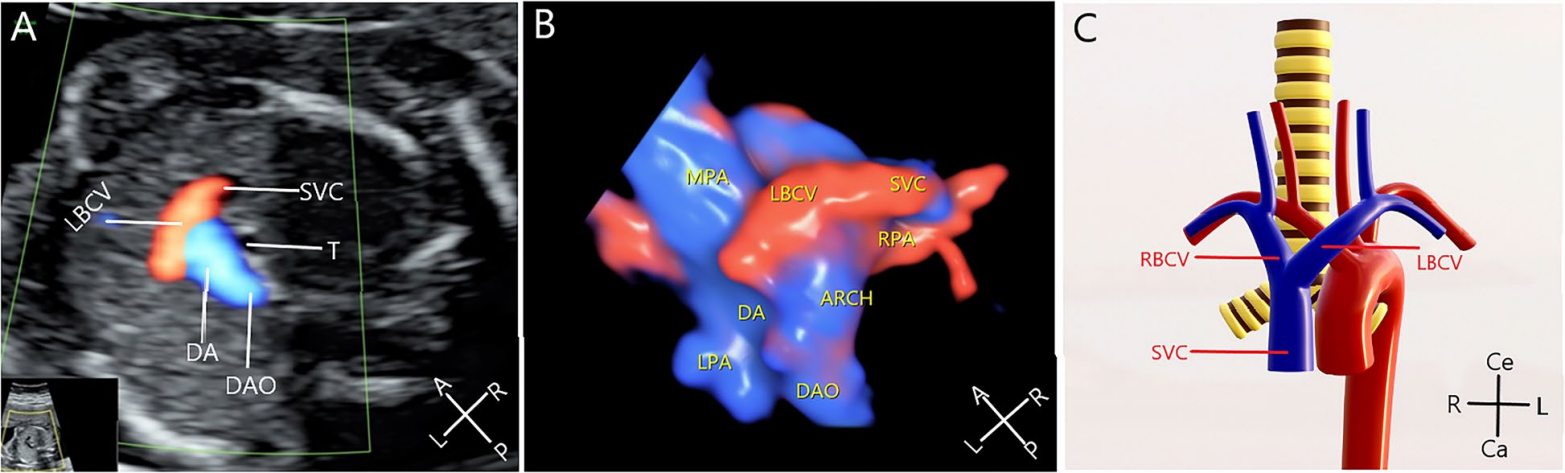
Images of the normal LBCV using two-dimensional (2D) and three-dimensional (3D) ultrasonography. A Color blood flow showing that the LBCV merges into the RSVC. B LBCV and RSVC are shown using HD-flow render mode and STIC. C Normal structural presentation of the LBCV and RSVC (anterior view). SVC, superior vena cava; DA, ductus arteriosus; DAO, descending aorta; RSVC, right superior vena cava; SP, spine, T, trachea; LBCV, left brachiocephalic vein; L, left; R, right; A, abdominal; P, spinal; Ce, cephalic; Ca, caudal
